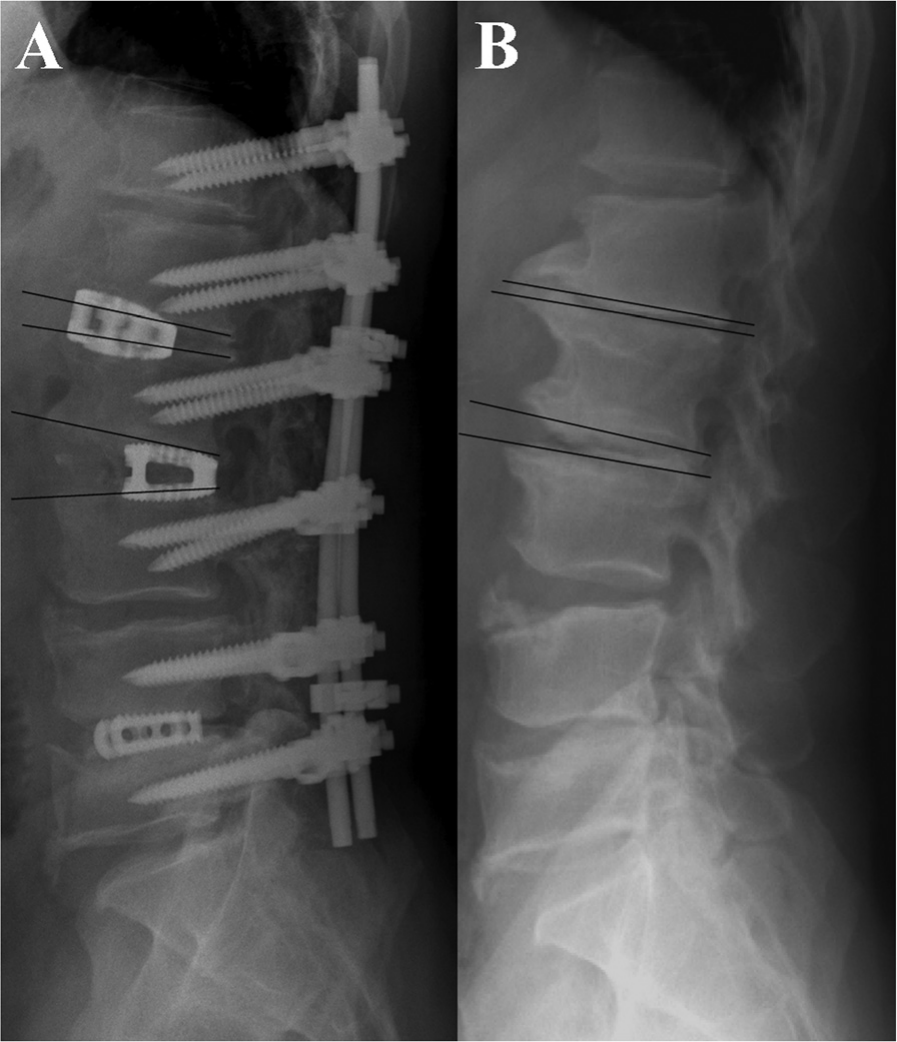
Radiographs of postoperative lateral view (A) and preoperative lateral view (B) showing a posteriorly placed L2-3 cage provided more sagittal plane correction (2° pre-op to 19° post-op, correction 17°) than a more anterior L1-2 cage (−2° pre-op to 4° post-op, correction 6°).
